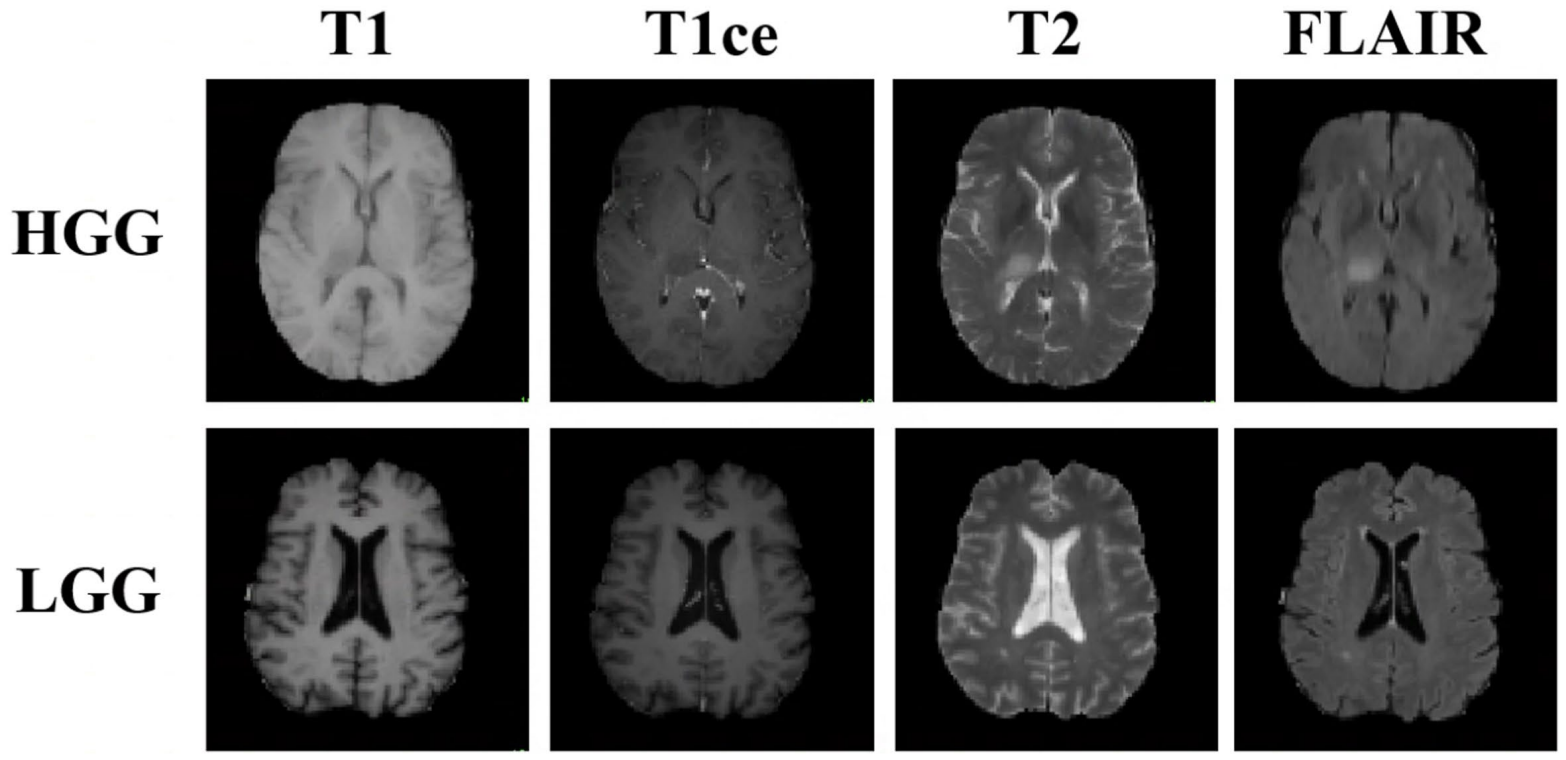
Examples of three-dimensional brain MR images of low-grade glioma (LGG) and high-grade glioma (HGG) samples. Each sample has images from four modalities: T1-weighted MRI, contrast-enhanced T1-weighted (T1ce) MRI, T2-weighted MRI, and fluid attenuation inversion recovery (FLAIR) MRI. These sequences provide complementary information for different subregions of brain tumors.
